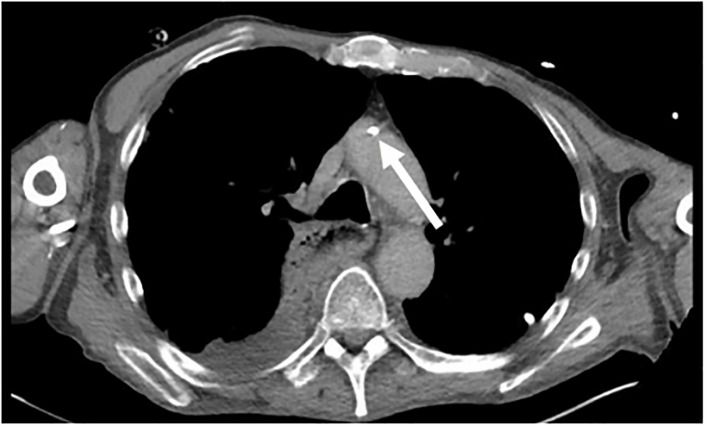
Axial view of a computed tomography angiogram depicting the central venous
